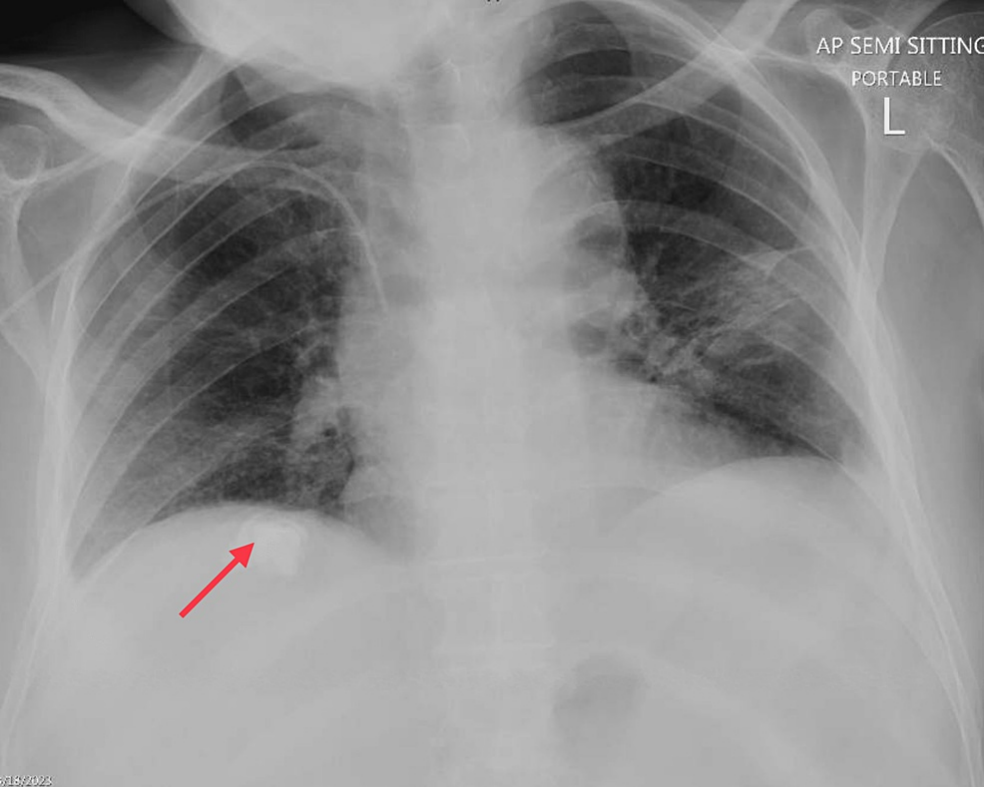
Chest X-ray showing the calcified lesion in the right upper quadrant (red arrow)
